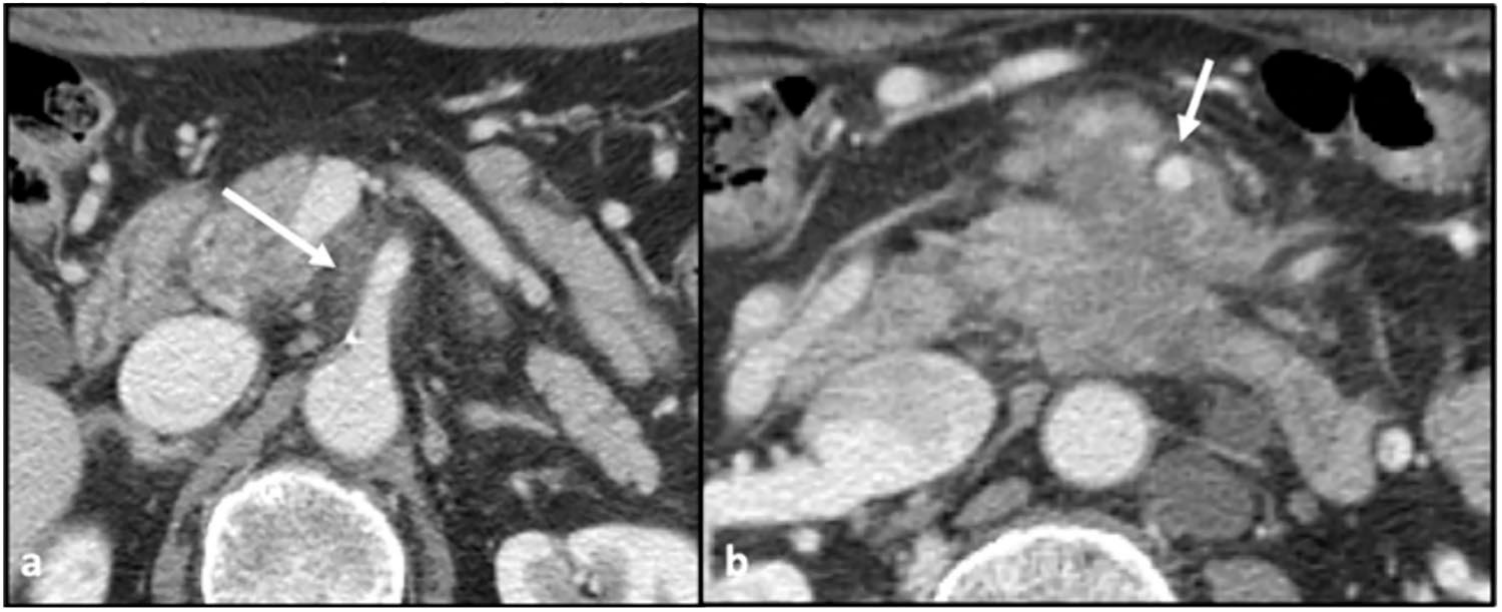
Axial CT section with portal venous phase contrast. a Infiltrative disease spread along the base of the small bowel mesentery, delineated by the superior mesenteric artery (arrow). b Disease spread along the more distal aspect of the superior mesenteric artery, in the jejunal mesentery (arrow)
